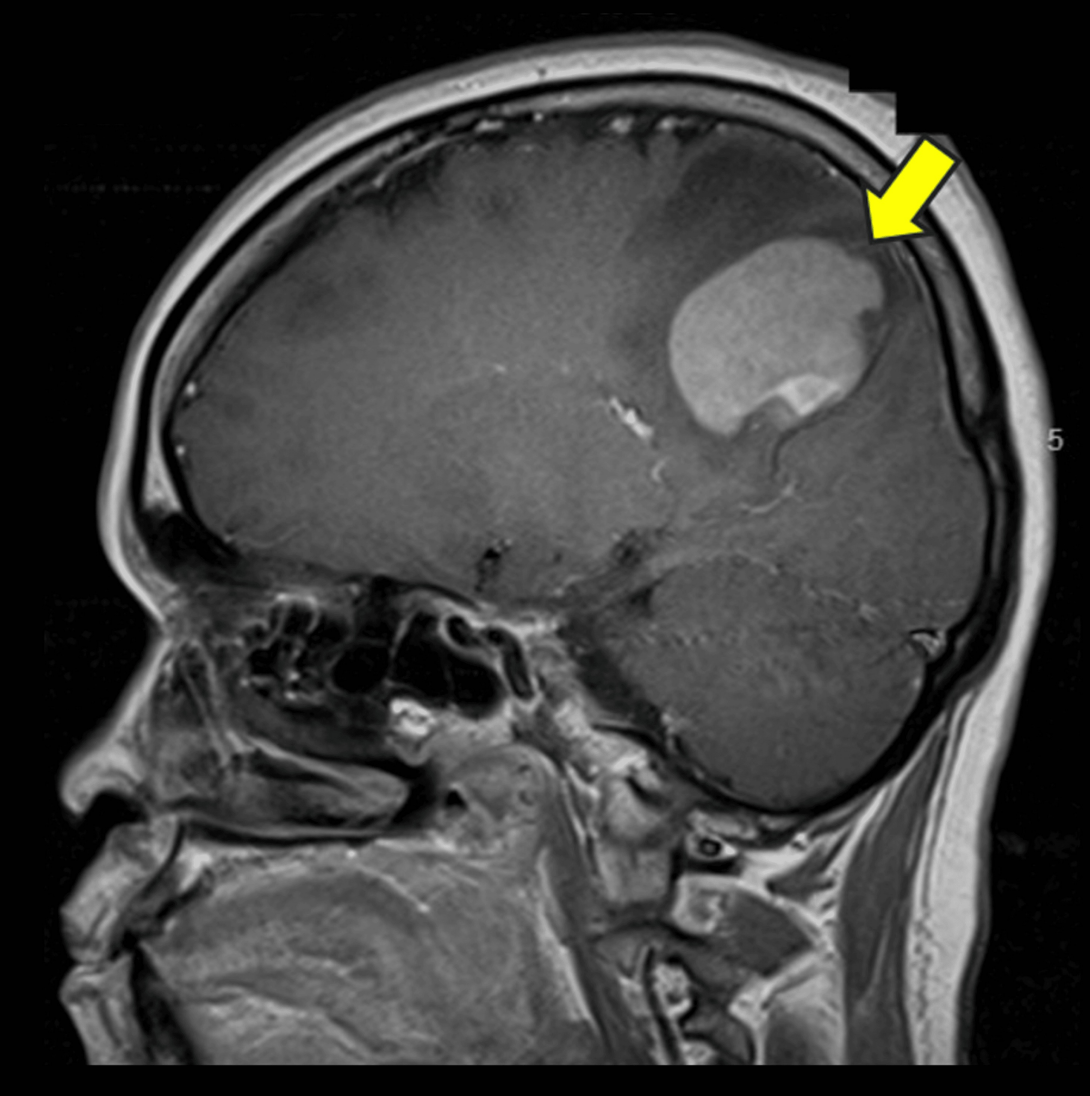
Sagittal T1-weighted contrast-enhanced MRI at presentation, showing a well-defined hyperintense intra-axial mass in the right parieto-occipital lobe (yellow arrow) at the parasagittal region. MRI: magnetic resonance imaging
